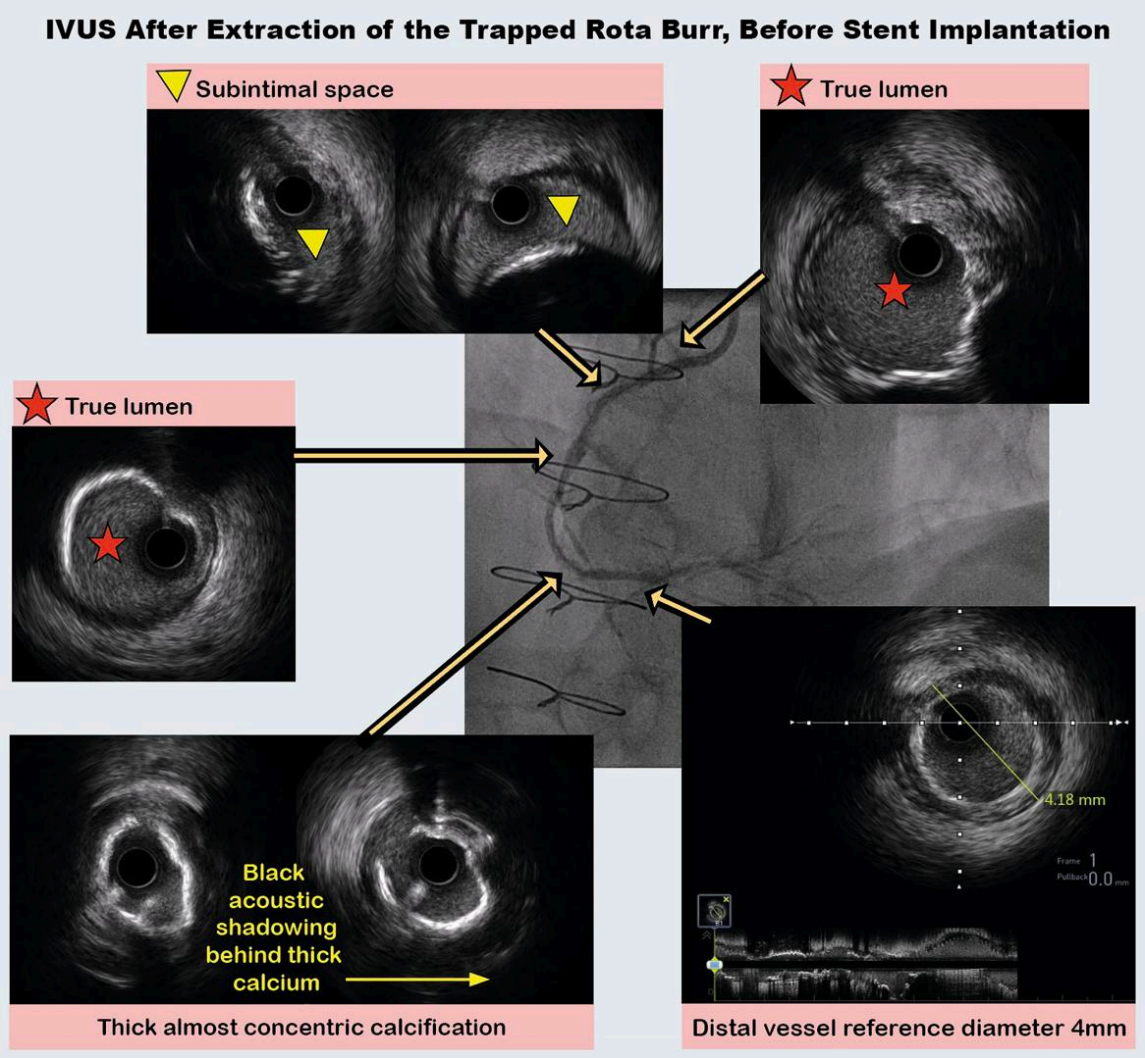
Intravascular ultrasound after burr extraction. IVUS, intravascular ultrasound.
